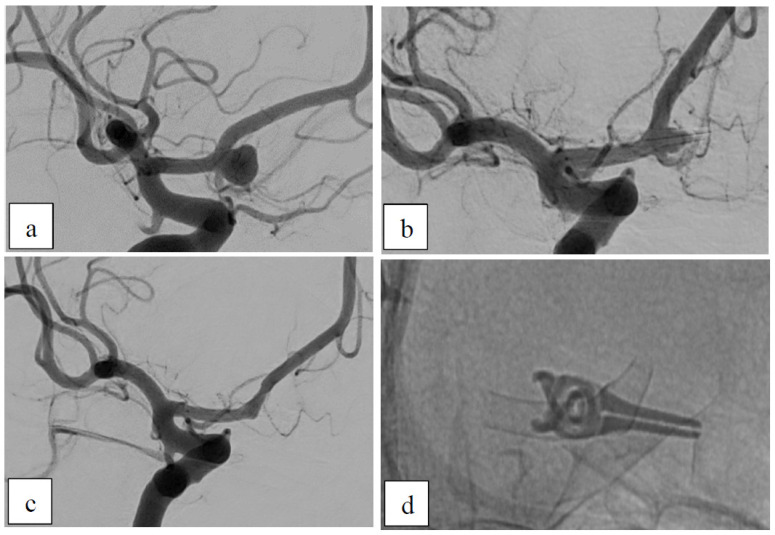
Recurrent aneurysm. (a) Right ICA angiogram showing the saccular aneurysm located at the AcomA. (b) Pre-interventional angiogram after clipping of the aneurysm and persisting inflow. (c) Post-interventional angiogram with complete occlusion of the aneurysm. (d) X-ray of the implanted FDS covered by the previously inserted clip.
